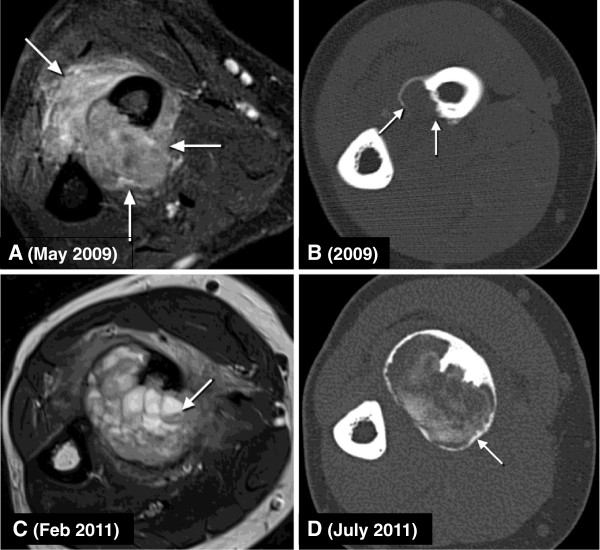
Imaging of the patients right forearm tumor. (A) Initial magnetic resonance imaging (MRI) demonstrating extensive involvement of the soft tissue between the radius and ulna as well as the cortex of the radius by an exclusively solid tumor mass (arrows). (B) Pre-treatment computer tomography (CT) scan with a small area of a split and disrupted cortex of the radius (arrows). (C) MRI directly prior to denosumab therapy with a locally progressive, extensive soft tissue mass following local surgical therapy 18 months previously. Fluid-fluid levels may be seen at this point (arrow). (D) CT scan following five months of denosumab therapy demonstrating almost complete containment of the soft tissue mass by a boney rim (arrow).
